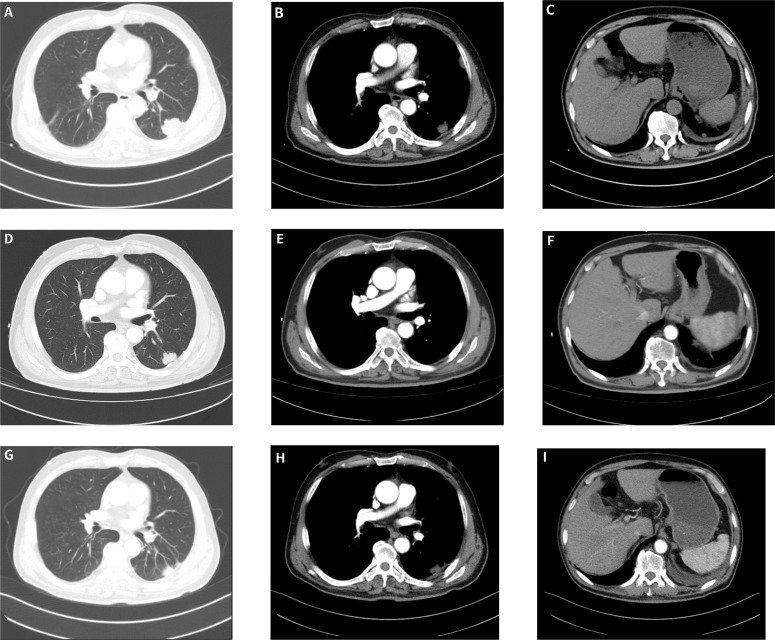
Representative chest computed tomography (CT) images following multiple cycles of treatment. (A) Lung window after 2 cycles of treatment; (B, C) Mediastinal window after 2 cycles of treatment. (D) Lung window after 4 cycles of treatment; (E, F) Mediastinal window after 4 cycles of treatment. (G) Lung window after 6 cycles of treatment; (H, I) Mediastinal window after 6 cycles of treatment.
